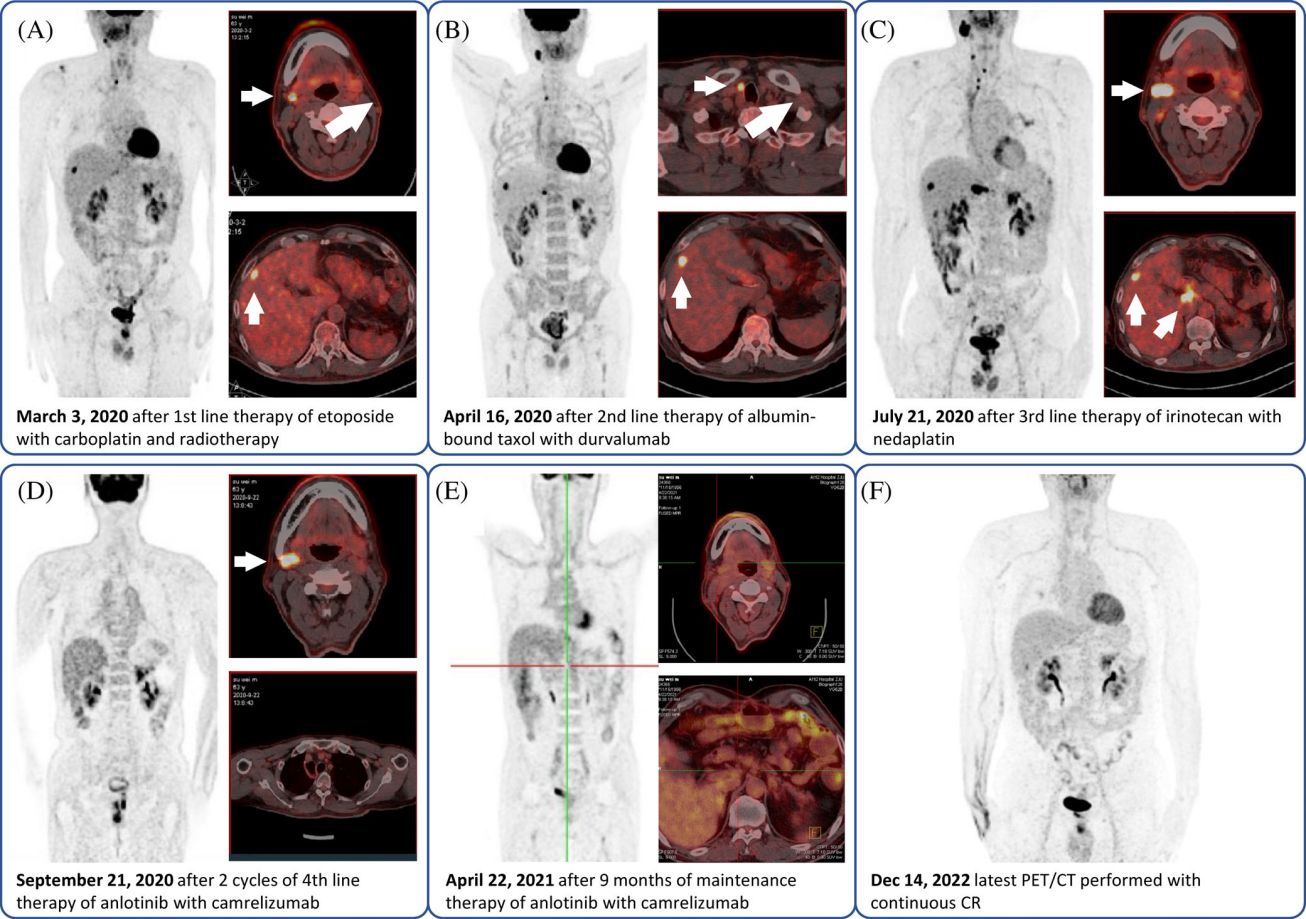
PET/CT images and treatment throughout the disease course after metastasis revealed in December 2019. (A) Metastasis in cervical lymph node and liver. (B) Metastasis in mediastinal lymph node and liver. (C) Metastasis in cervical lymph node, liver and retroperitoneal lymph node. (D) Metastasis detected only in cervical lymph node. (E, F) No metastasis detected.
